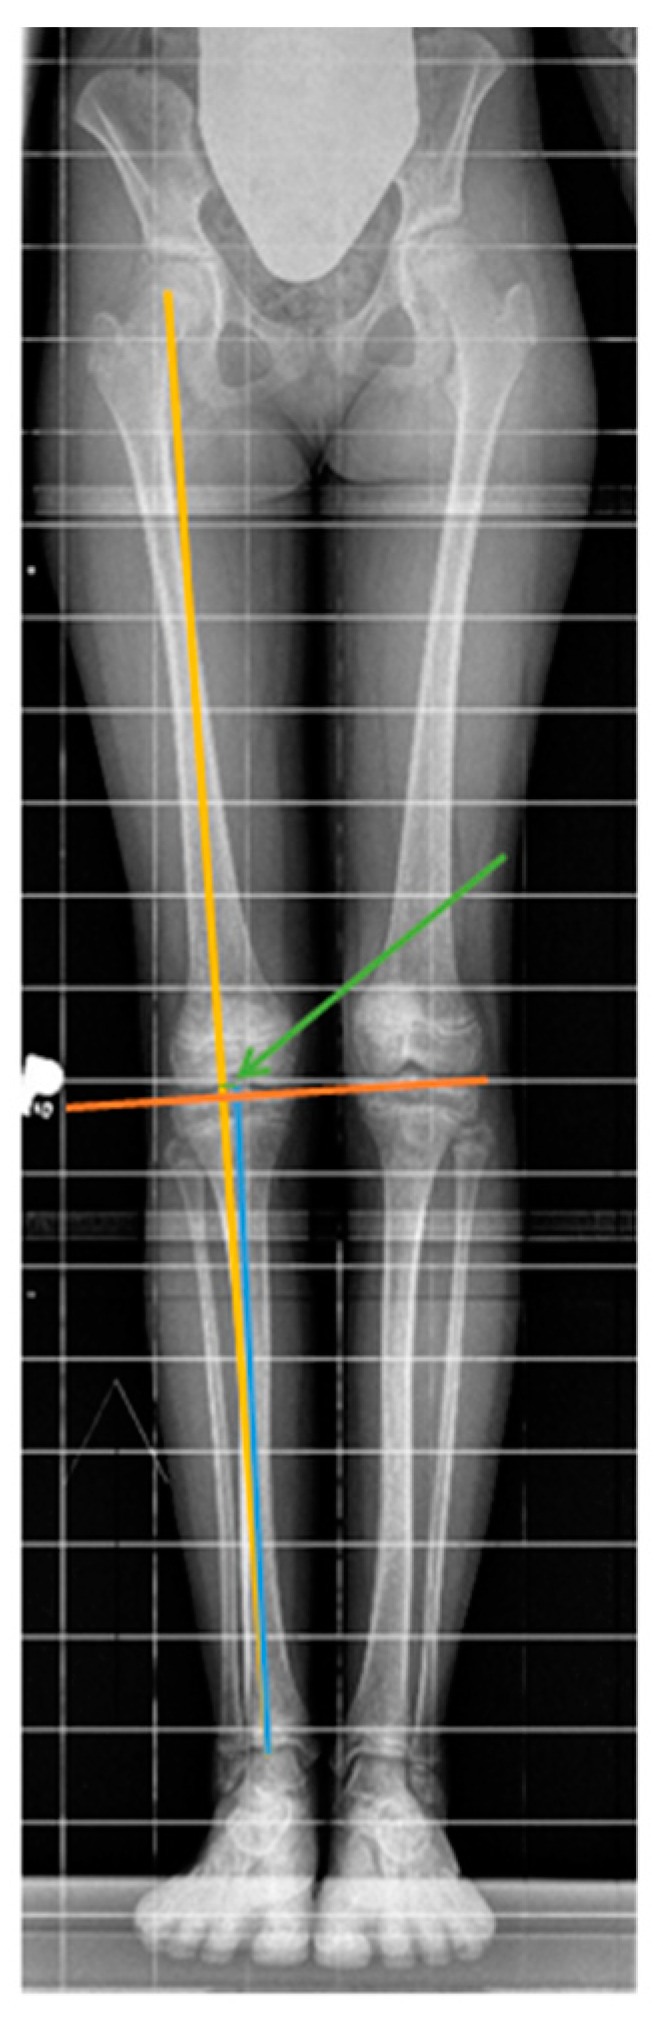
A standing X-ray image of a 10-year-old girl with leg length discrepancy (LLD) taken prior to epiphysiodesis. Graphic image of the M/aT (angle between the mechanical axis of the limb—yellow line, and the anatomical axis of the tibia—blue line), mMPTA (angle between the mechanical axis of the limb—yellow line, and the tibial joint line—orange line), and MAD (deviation of the mechanical axis from the center of the knee joint—medial or lateral—represented by a green line and indicated by a green arrow).
